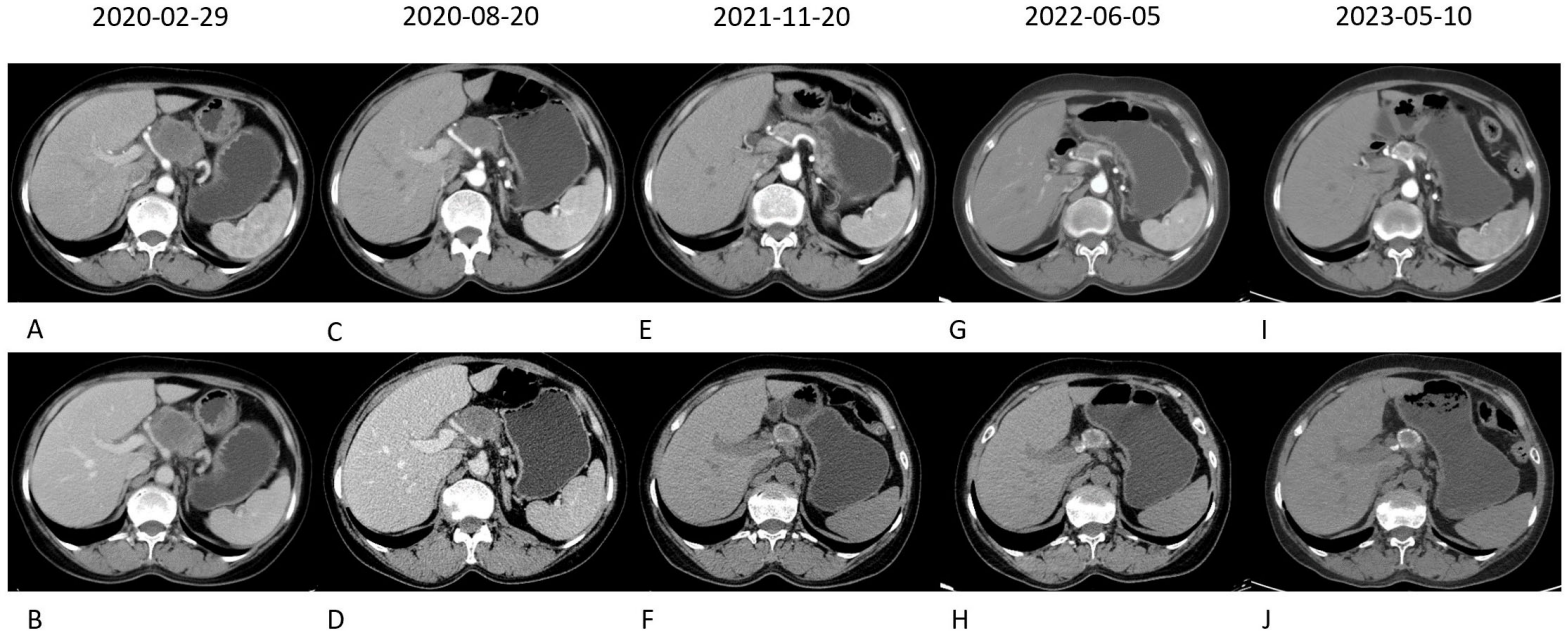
Contrast-enhanced plain CT showed the mass in close contact with the surrounding blood vessels (A, B). After 2 months of targeted immunotherapy, the tumor was slightly reduced, and the relationship with the surrounding blood vessels was clearer (C, D). After conservative treatment for more than 1 year, the mass was significantly reduced and a ring of high-density shadow appeared around it, which was considered to form a calcification package (E–H). The size of the mass remained the same, whereas the density of the annular calcification layer increased (I, J).
